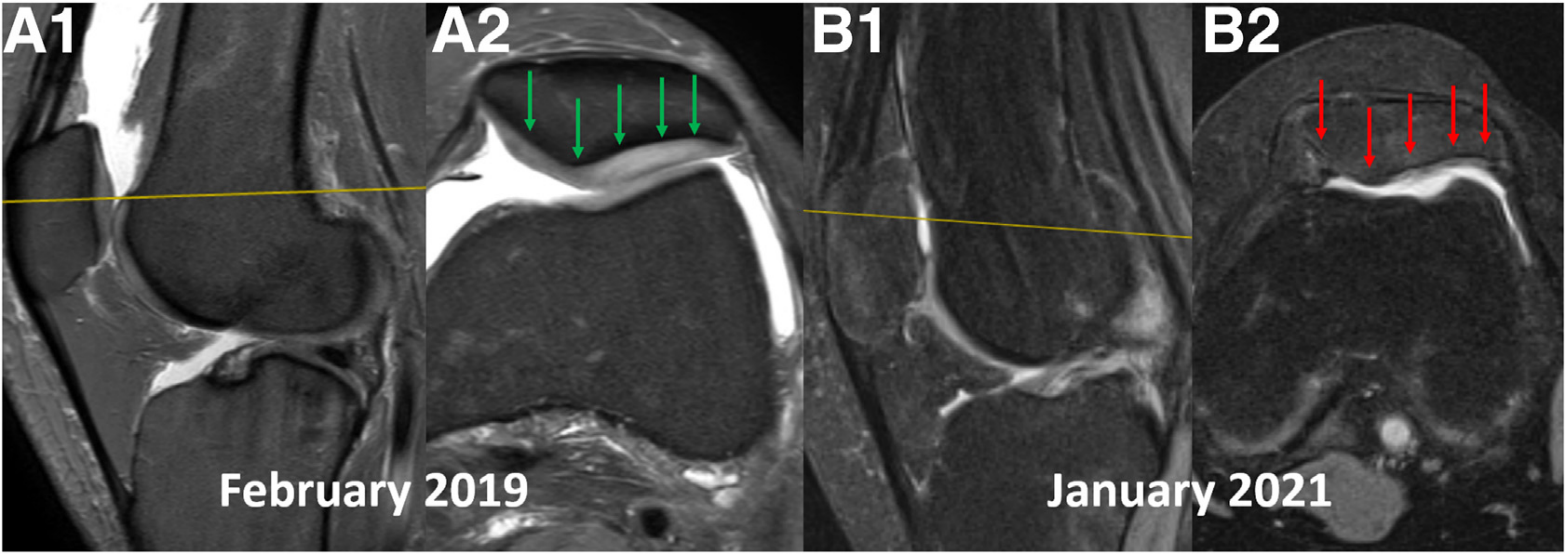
Severe patellofemoral cartilage damage in a relatively short time in a patient with knee extension contracture, due to overload during rehabilitation by means of painful, excessively forceful flexion. The left knee, sagittal (A1 and B1) and axial (A2 and B2) MRI scans. The patient, who has a knee extension contracture, was rehabilitated by means of painful, excessively forceful flexion. While the improvement in knee flexion was minimal, the patellofemoral cartilage (arrows) was severely damaged in a relatively short time (February 2019 to January 2021) as a result of these manipulations.
